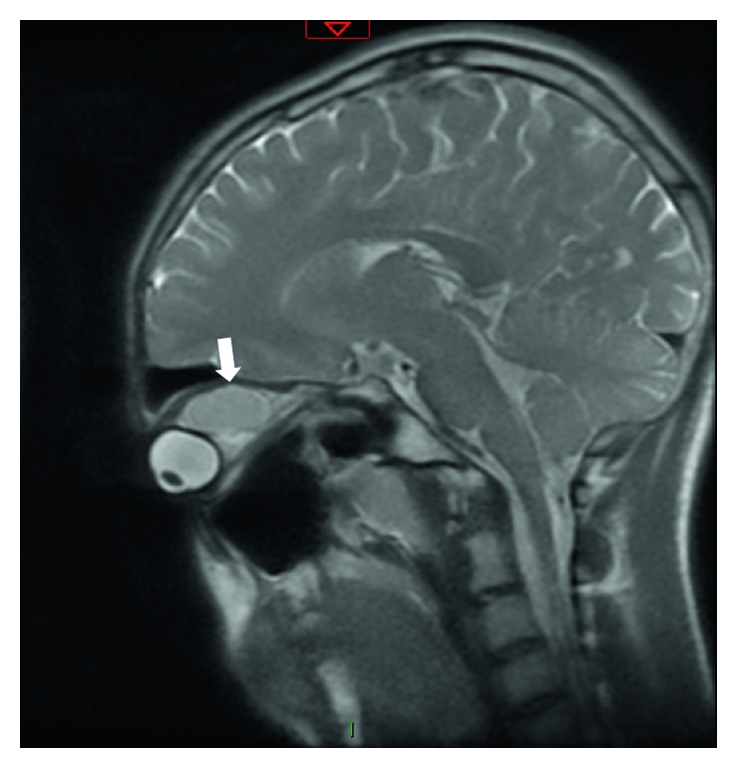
Sagittal T1-weighted MRI. The exact position and extent of the tumor and its relation to the optic nerve are clearly visible. There is a noticeable ocular bulb dislocation (white arrow).
